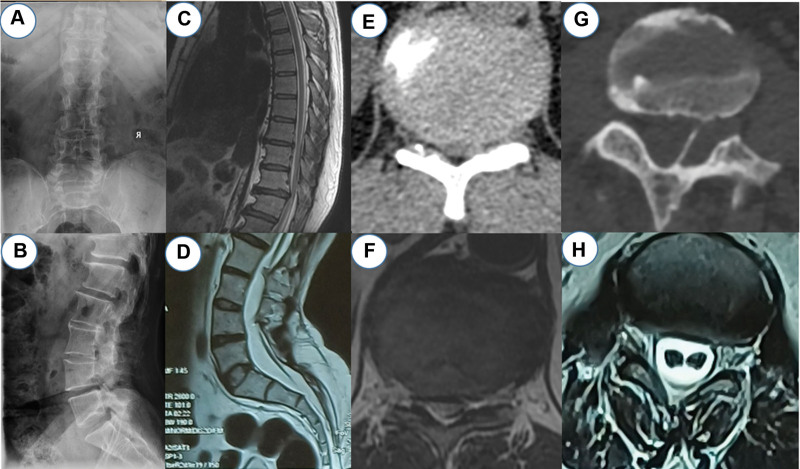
Imaging examinations performed prior to surgery. Anteroposterior (A) and lateral plain (B) radiographs of the lumbar spine. T2-weighted sagittal MRI of the lumbar spine showed an abnormal herniated disc at the T11-12 level and a low conus medullaris at the L4 level. (E and F) CT and axial MRI of the thoracic spine confirmed thoracic disc herniation and spinal stenosis at T11/T12. (G and H) CT and axial MRI of the lumbar spine show the bony structure splitting the cord into 2 hemi cords. CT = computed tomography, MRI = magnetic resonance imaging.
